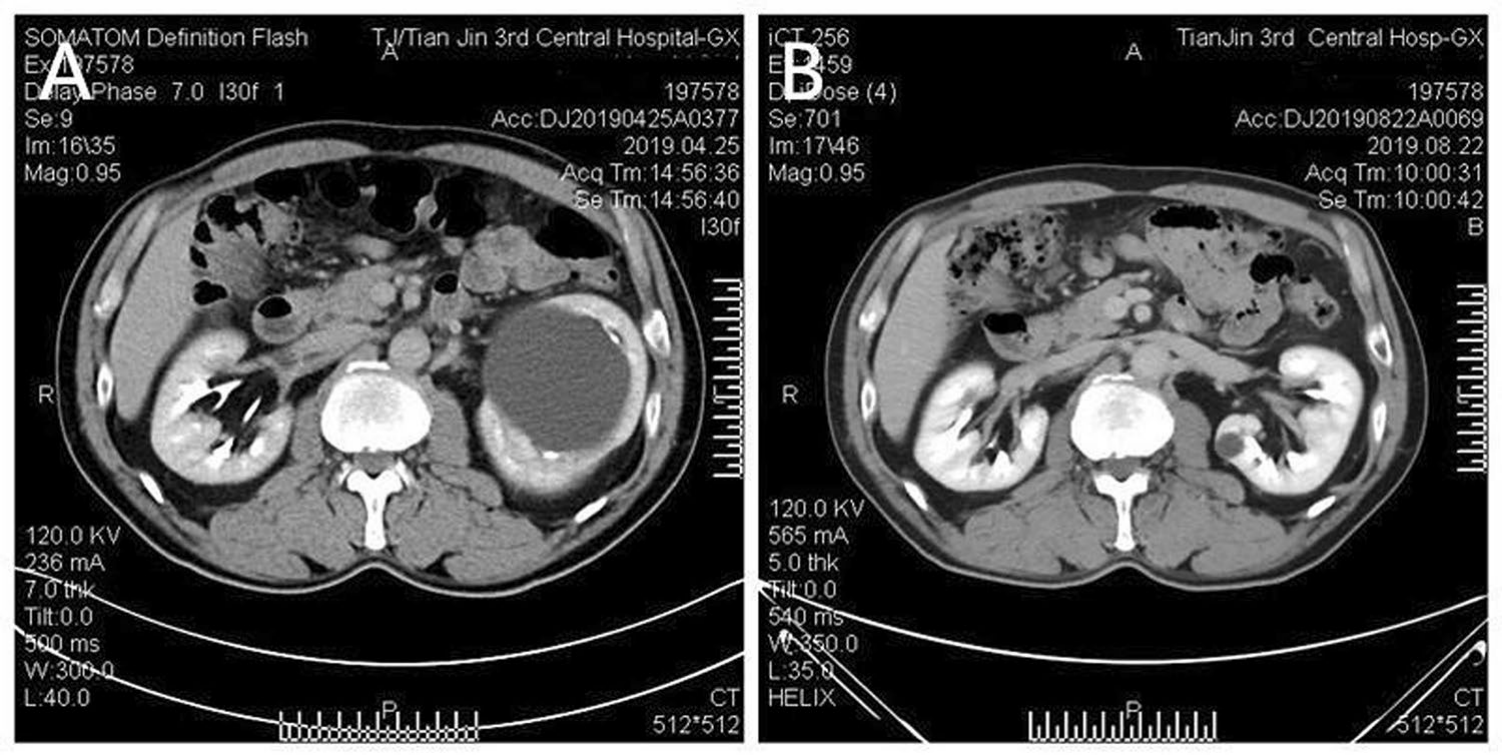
Preoperative and postoperative computed tomography. (A) Preoperative computed tomography image of the left renal parapelvic cyst. (B) Postoperative computed tomography, 3 months after surgery, showed the cyst had disappeared.
